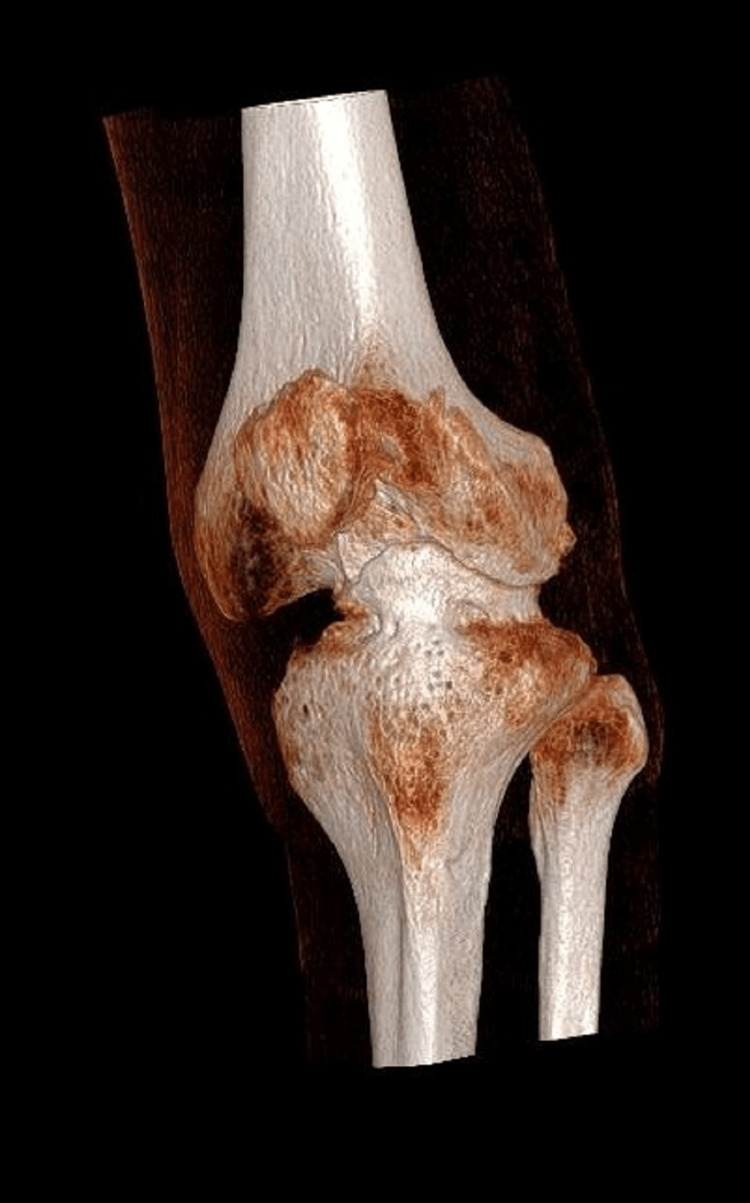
Preoperative CT reconstruction of the left knee. Significant bone loss is seen on the tibial plateau and the femoral condyles.
